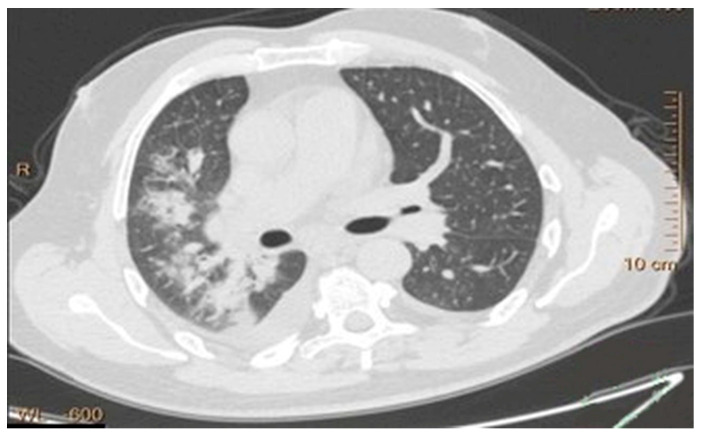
Lung cancer nodules in CT scans.
